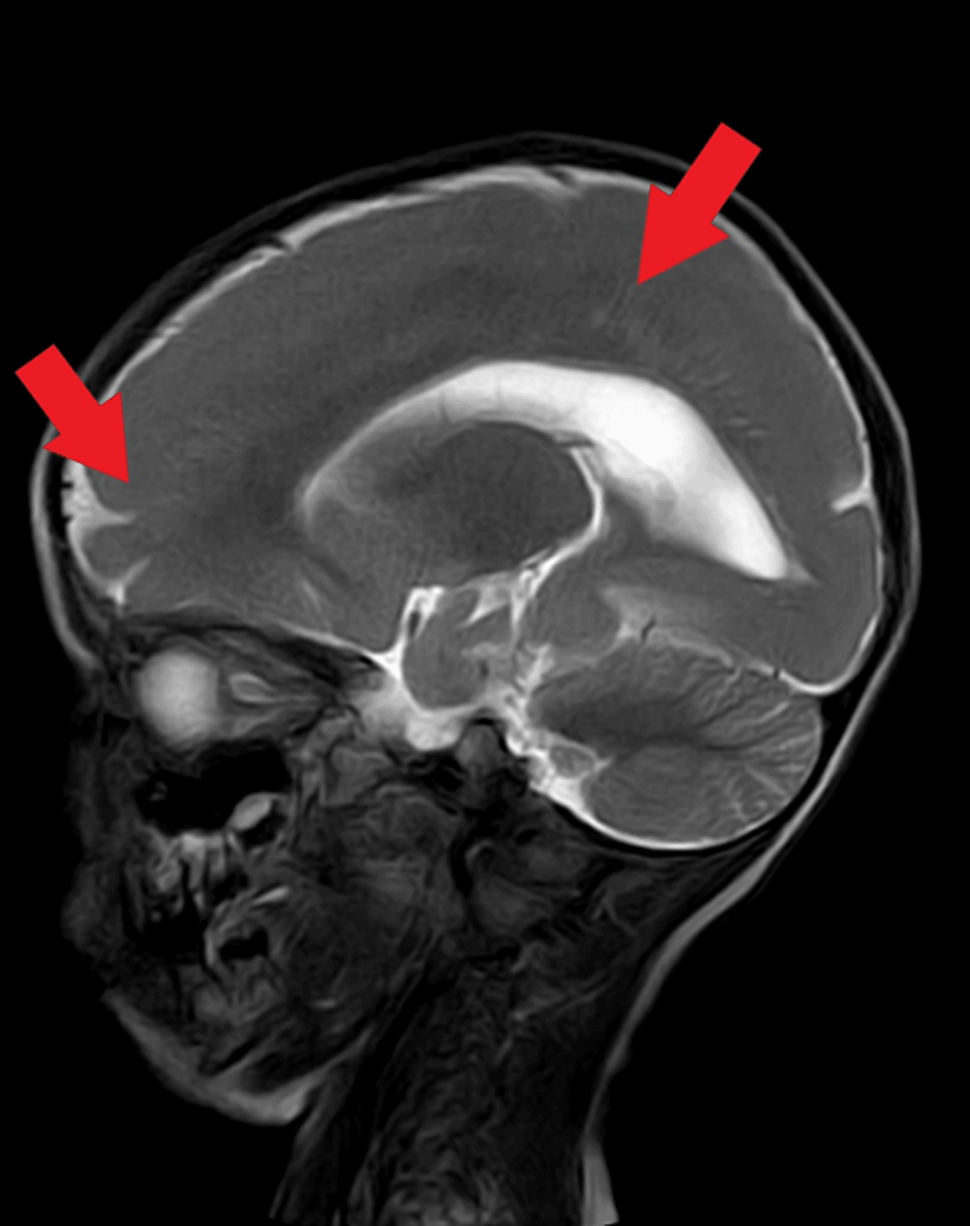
Magnetic resonance image demonstrating smoothing of sulci and gyri spaces in the brain, typically suggestive of type 1 lissencephaly
